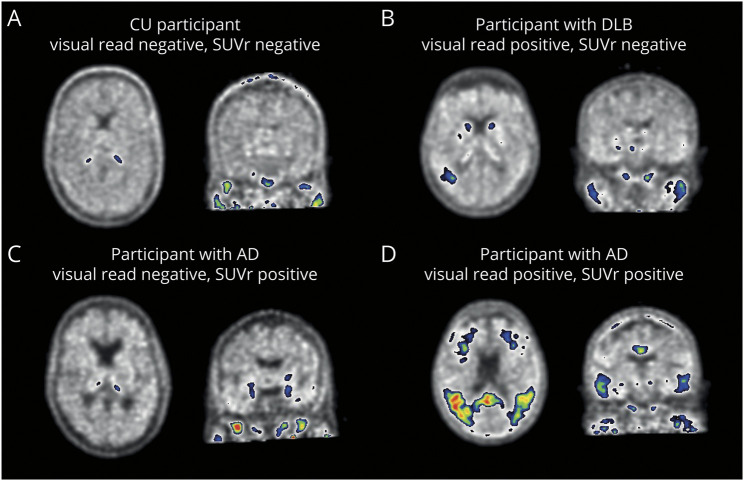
Example [^18^F]Flortaucipir PET Scans for Visual Read Shown are [^18^F]flortaucipir PET scans of 4 participants. (A) A CU participant defined as tau-negative on both visual read and SUVr. (B) A participant with DLB defined as visual read tau-positive, but SUVr negative. Increased tracer uptake was observed in only a small region, potentially resulting in low SUVr. (C) A participant with AD defined as visual read negative, but SUVr positive. Increase tracer uptake was observed isolated to the medial temporal lobe, which does not contribute to a positive tau-PET visual read. (D) A participant with AD defined as tau-positive on both visual read and SUVr. AD = Alzheimer disease; CU = cognitively unimpaired; DLB = dementia with Lewy bodies; SUVr = standardized uptake value ratio.
